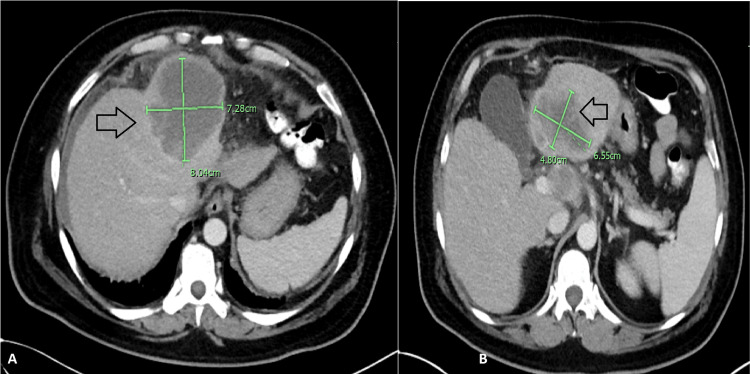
January 2020 CT scan of the abdomen Radiological evidence of progression in both left hepatic lobe masses is seen (arrows in A and B)
